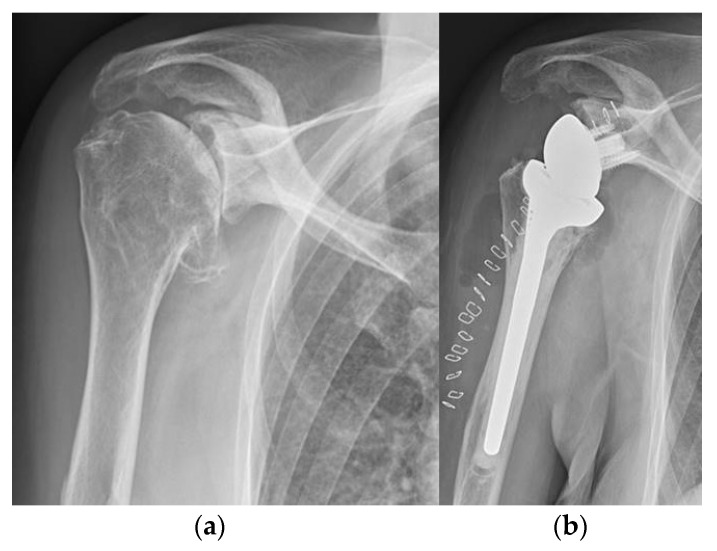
Reverse total shoulder arthroplasty (rTSA). (a) preoperative X-ray; (b) postoperative X-ray after reverse total shoulder arthroplasty.
